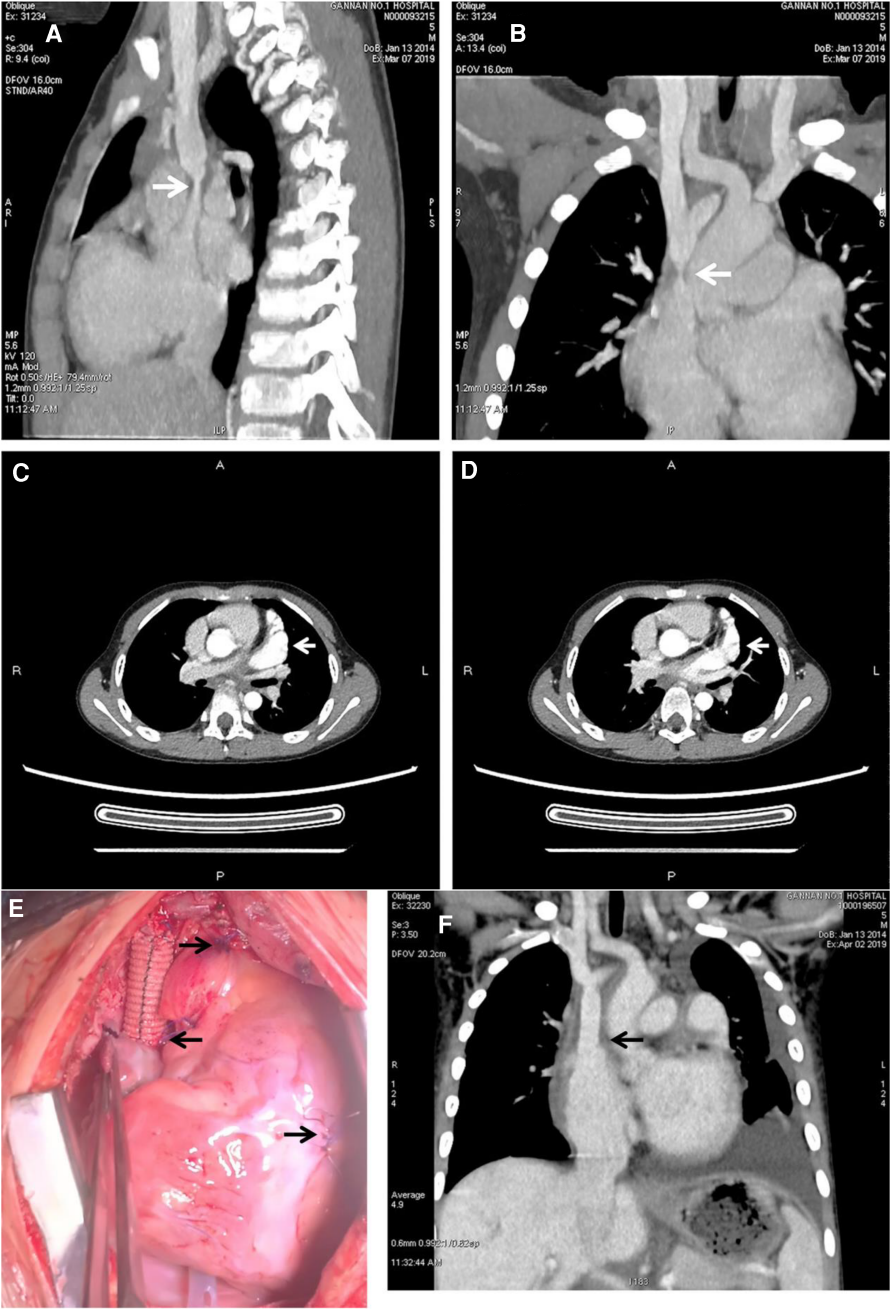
Preoperative CT and CTA showed the stenosis of the superior vena cava and left atrial appendage aneurysm. Intraoperative and postoperative CT showed the reconstruction of the superior vena cava with artificial blood vessels. (A,B) Showed severe stenosis at the junction of superior vena cava and right atrium (arrowhead). (C,D) Showed that the enhancement of the left atrial appendage was consistent with that of the ascending aortic cavity, and the arrowhead referred to the enlarged left atrial appendage (arrowhead). (E) Showed the details of the operation; the left arrow showed the site of anastomosis between the artificial vessel and the right atrium; the first arrow on the right showed the site of ligation and suture at the root of the left atrial appendage; and the second arrow on the right showed the site of the temporary pacemaker. (F) Showed that the blood flow between the artificial vessel and the right atrium was unobstructed, which relieved the stenosis of the superior vena cava.
